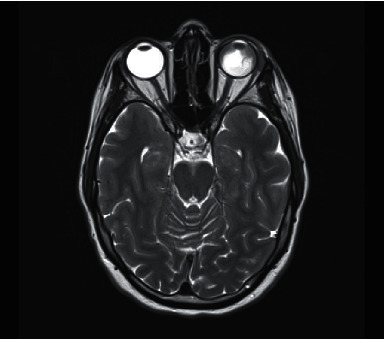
Exudative retinal detachments and thickening of globe tissue seen on MRI orbits (T2 sequence).
